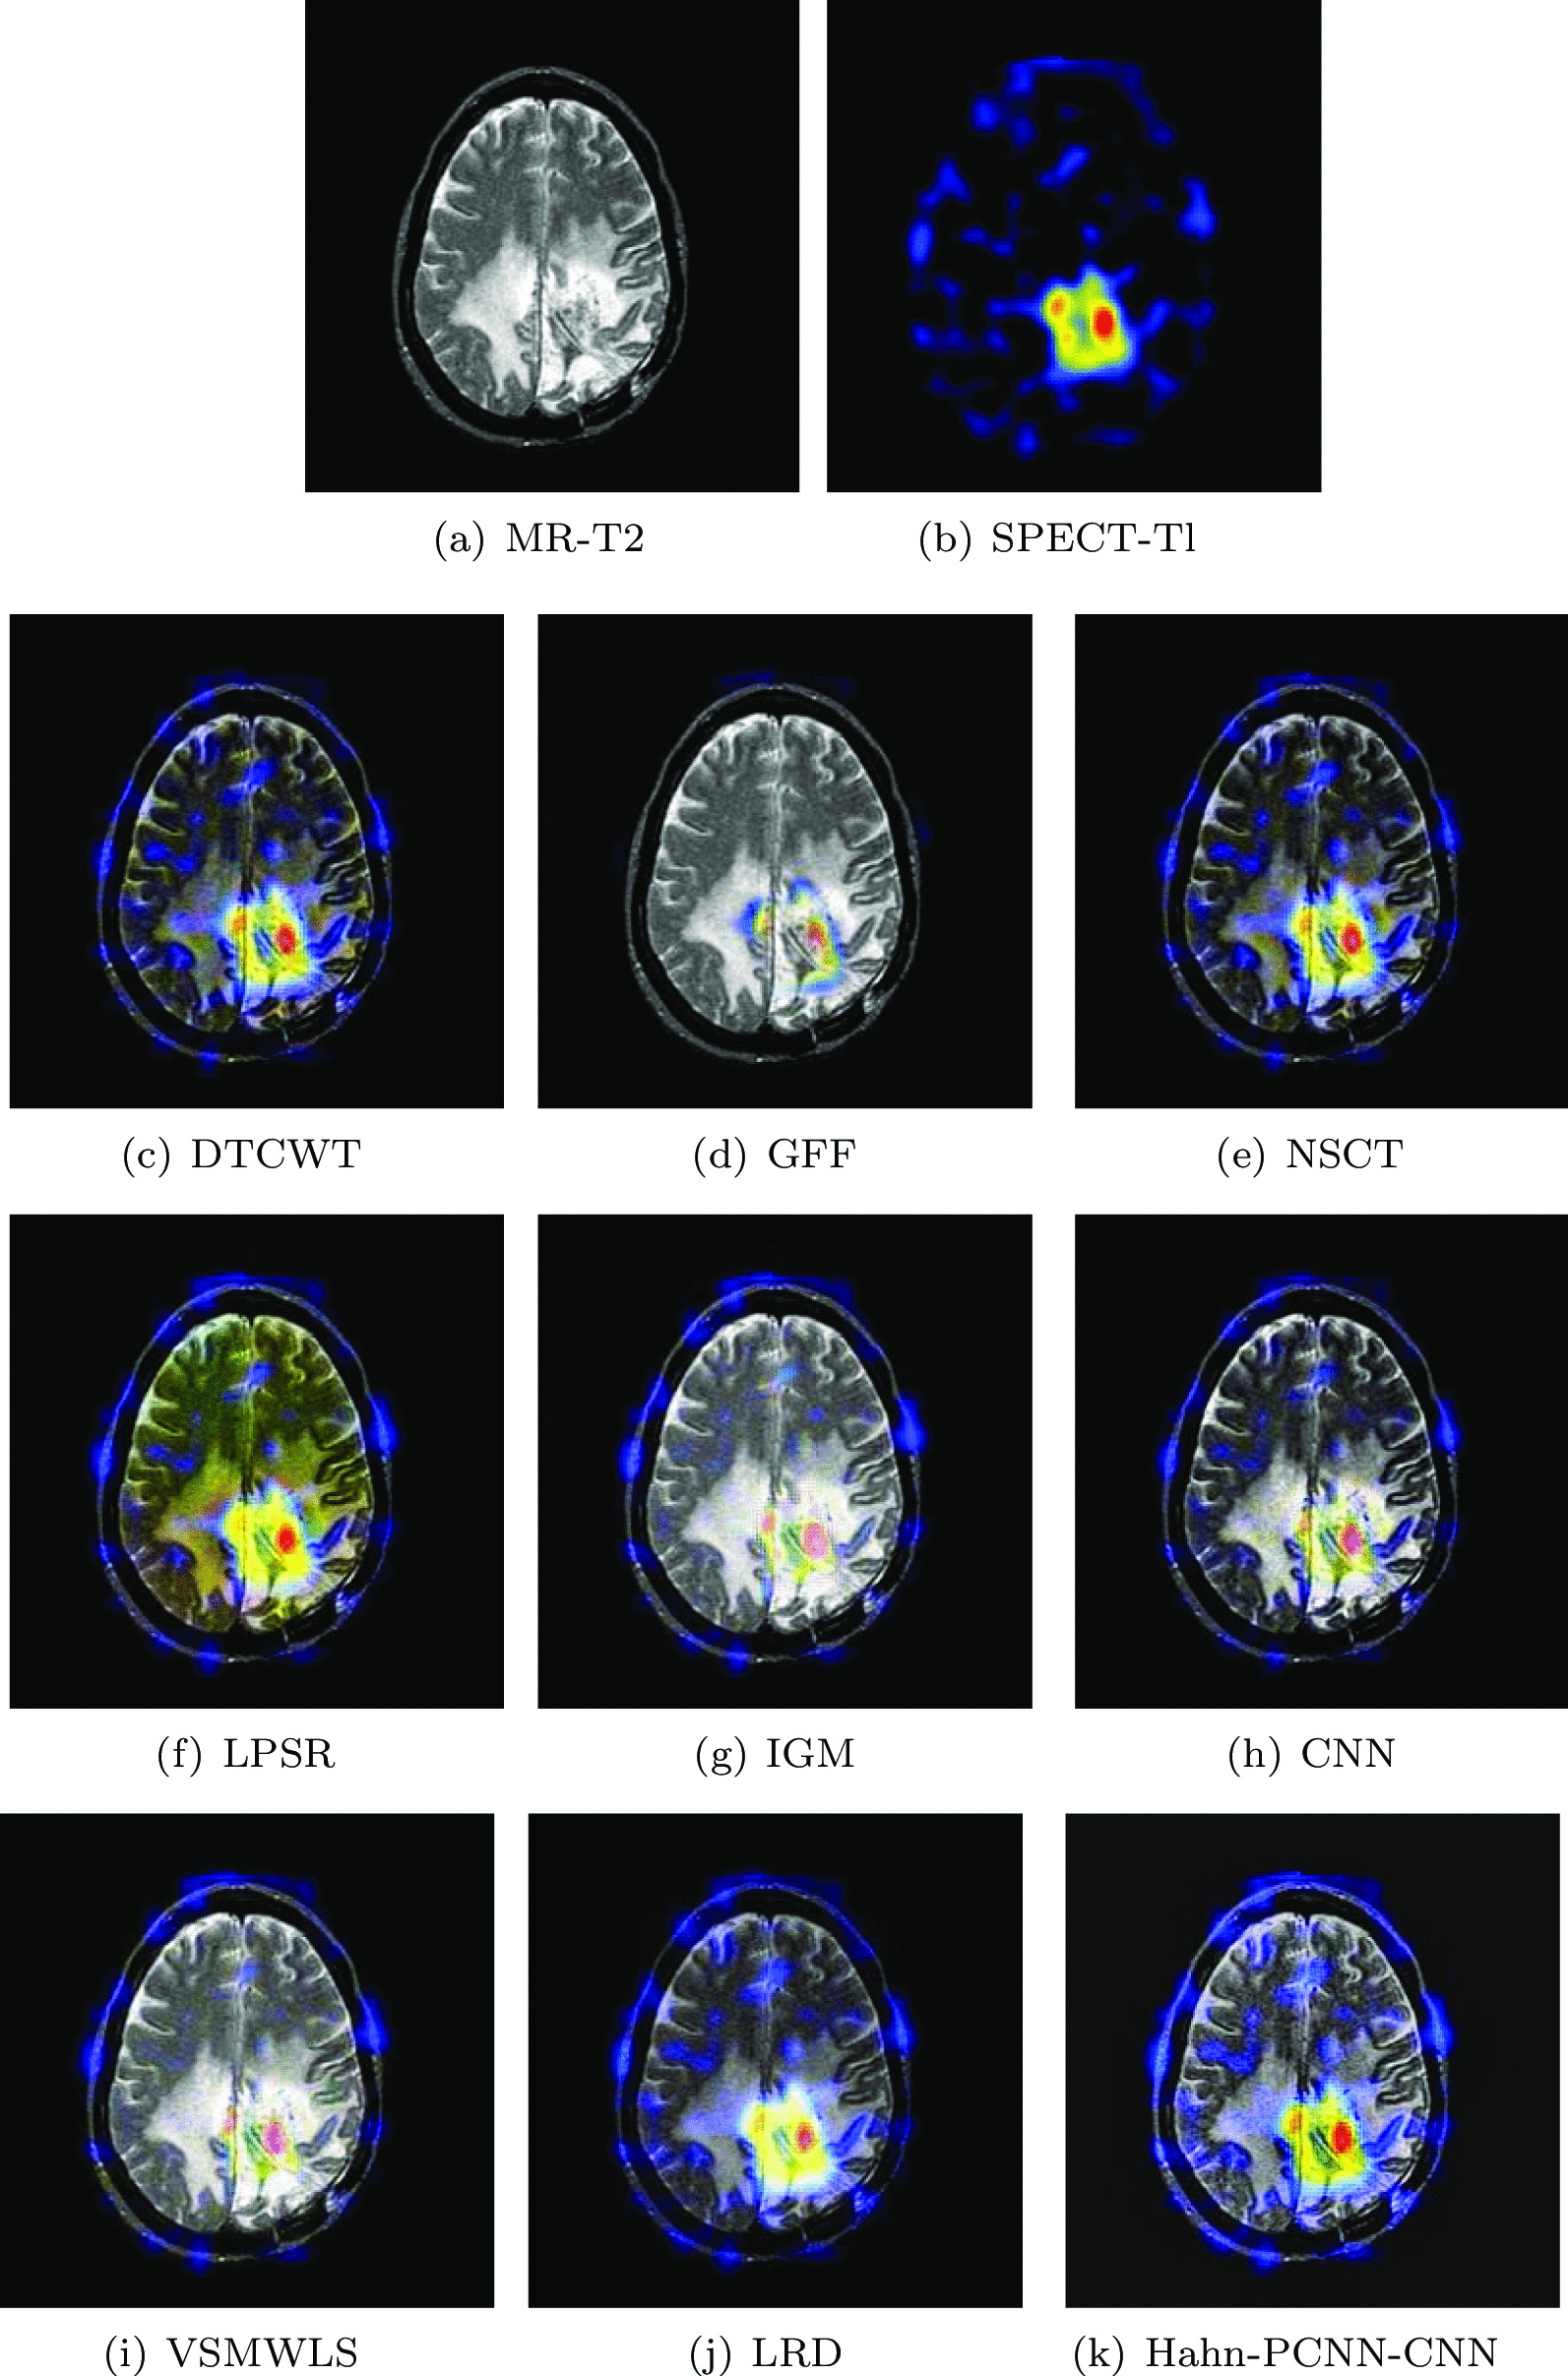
The second set of fused MRI-SPECT images from 9 methods on glioma
